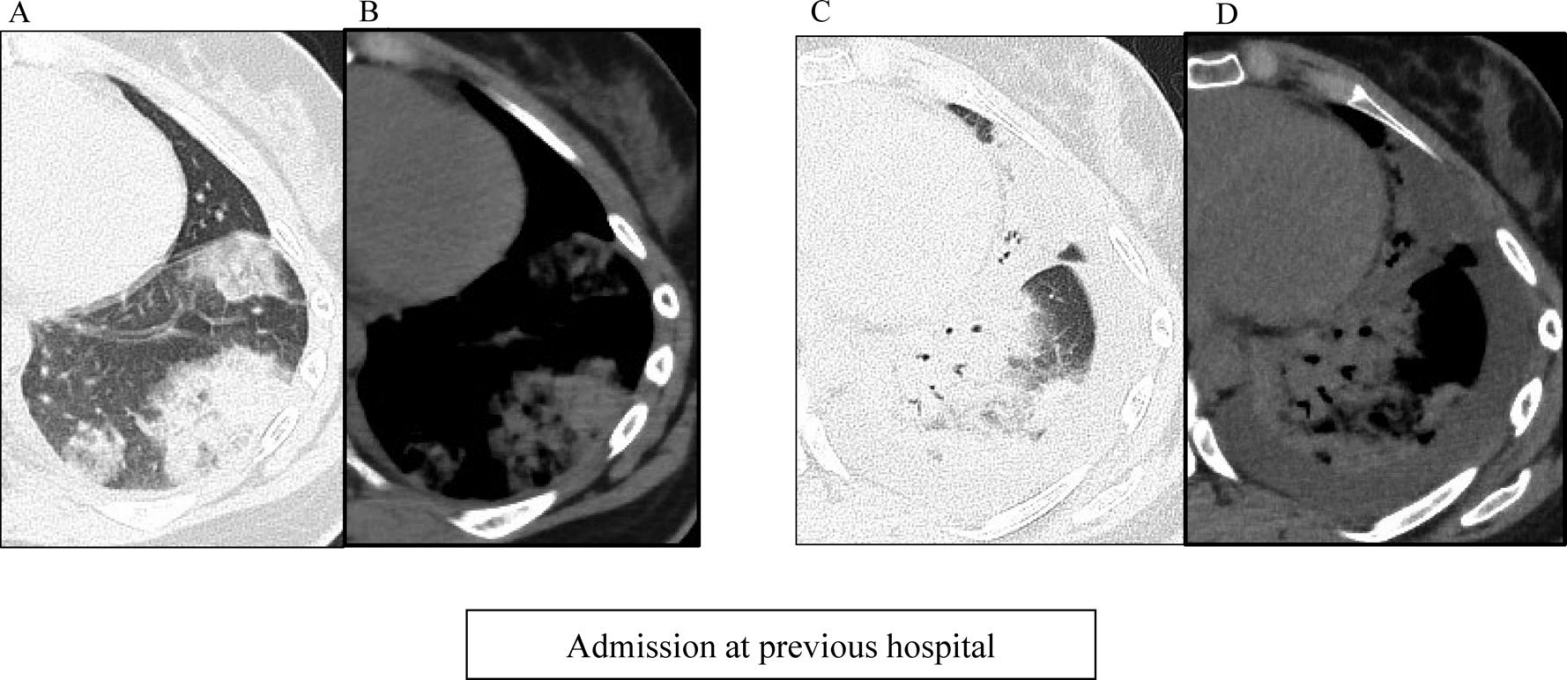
Plain computed tomography images obtained at the previous hospital. (A and B) Images obtained on the first day of admission at the previous hospital. (A) Lung window setting. Poorly demarcated patchy consolidation is seen in the left lower lobe. (B) Mediastinal window setting. Mild pleural effusion is seen. (C and D) Images obtained on the fourth day of admission at the previous hospital. (C) Lung window setting. (D) Mediastinal window setting. Pleural effusion has increased leading to atelectasis of the left lower lobes
